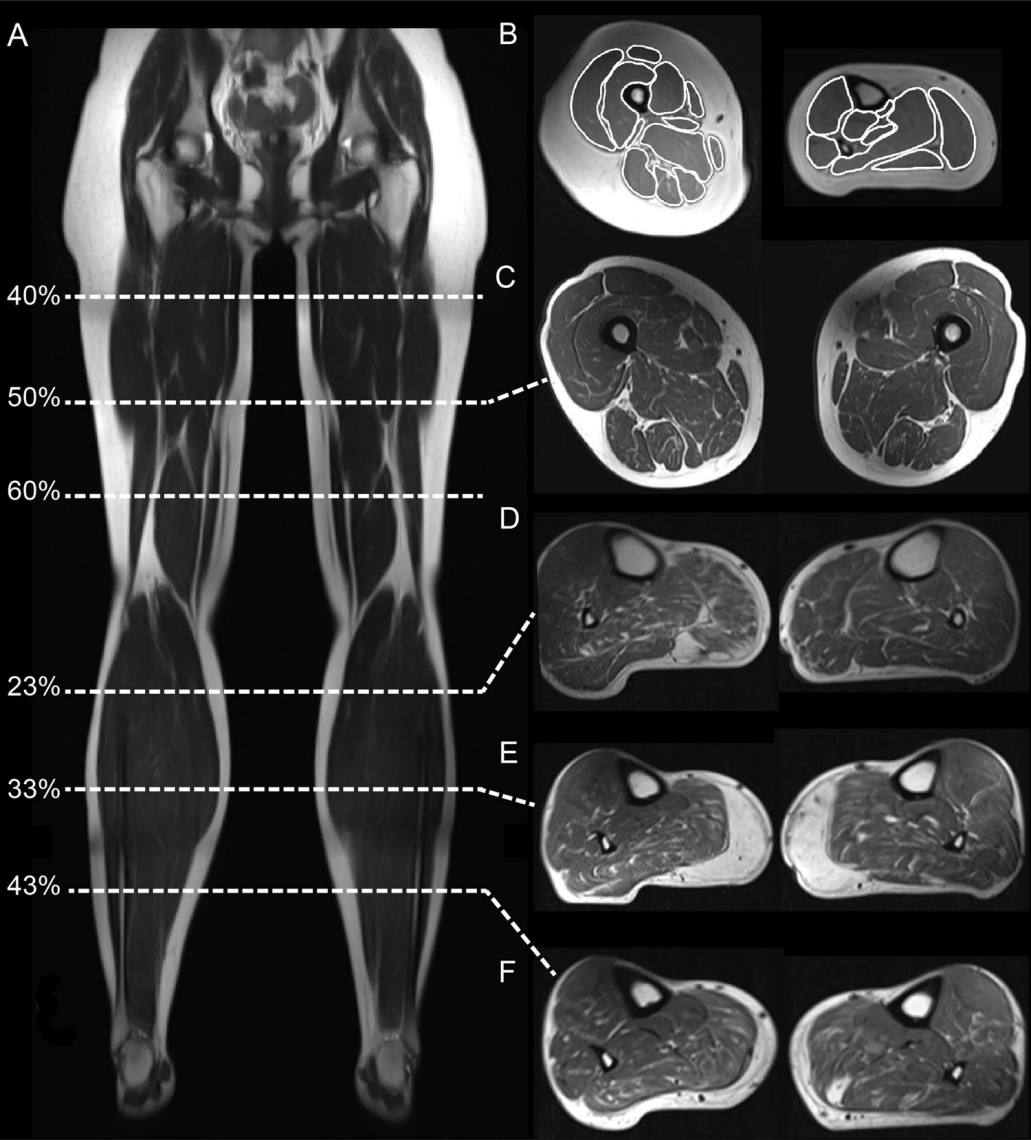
Overview of MRI. (A) Localizer shows the 6 different levels of segmentation on the leg. (B) Example of muscle mapping of the thigh (50%) and calf (33%). (C) T1-w MRI of a 59-year-old patient heterozygous for p.T1313M at 50% length of the thigh. (D–F) Series of T1-w MRI from the same patient at 23, 33 and 43% of the calf, demonstrating significant proximal–distal difference of involvement. In the more distal part of the muscle (at 33% length of the tibia), the gastrocnemius medialis is almost completely replaced by fat.
